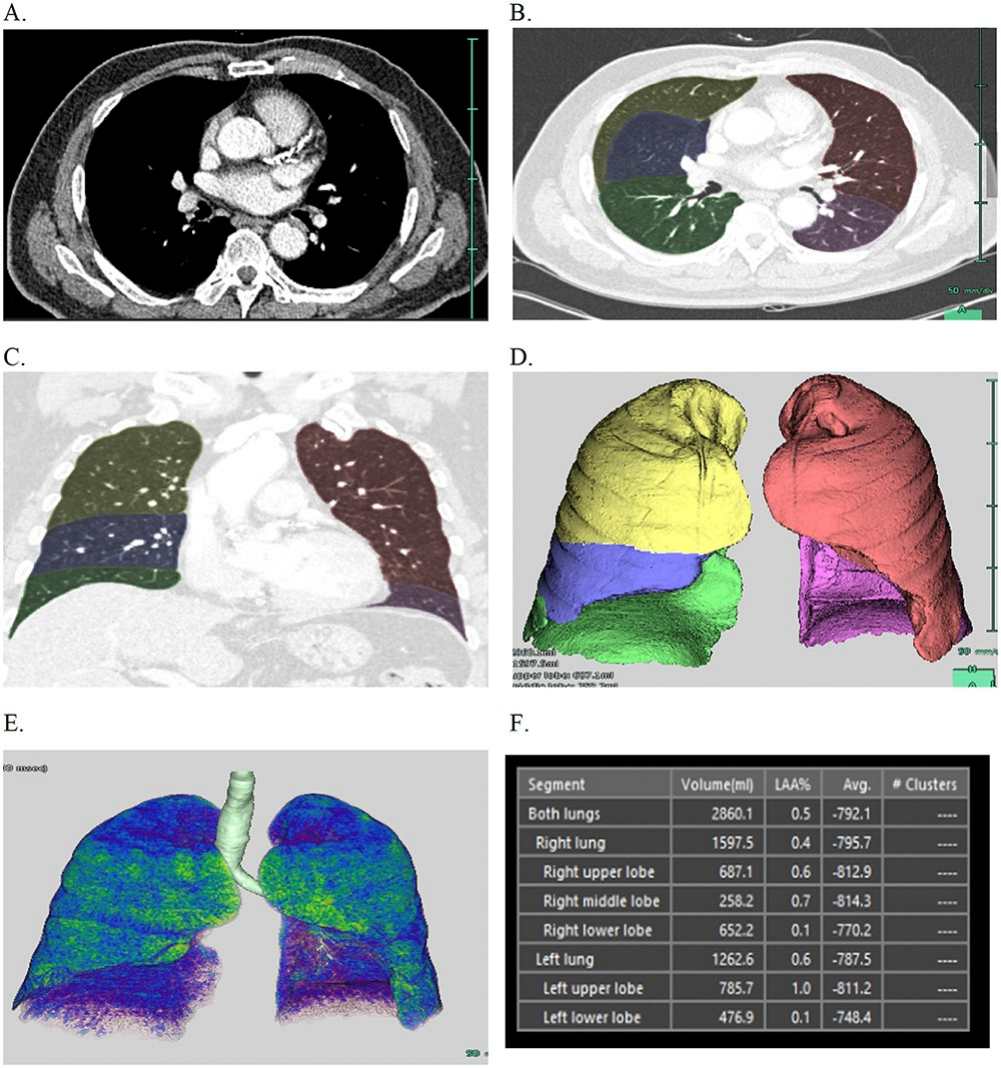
Process of obtaining emphysema index and lobe-specific emphysema index. Computed tomography image (A) is converted to a three-dimensional volume rendering image using a commercially available software, Synapse 3D Vincent^™^. Lobes are divided via the automatic segmentation technique and manual correction (B, C), and the volume of each lobe is produced (D). Lobe-specific emphysema index for each lobe is obtained by defining the emphysema index with an attenuation value lower than -950 Hounsfield unit (E, F).
